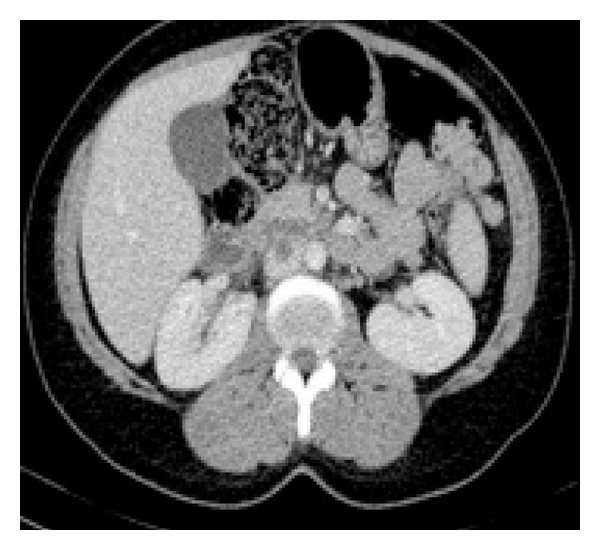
Axial CT; thrombosis in IVC.
